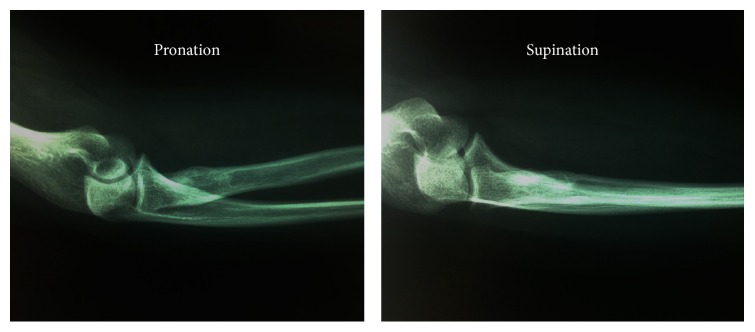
Radiograph images of the patient's elbow in pronation and supination, 8 years postoperatively with no signs of recurrence.
